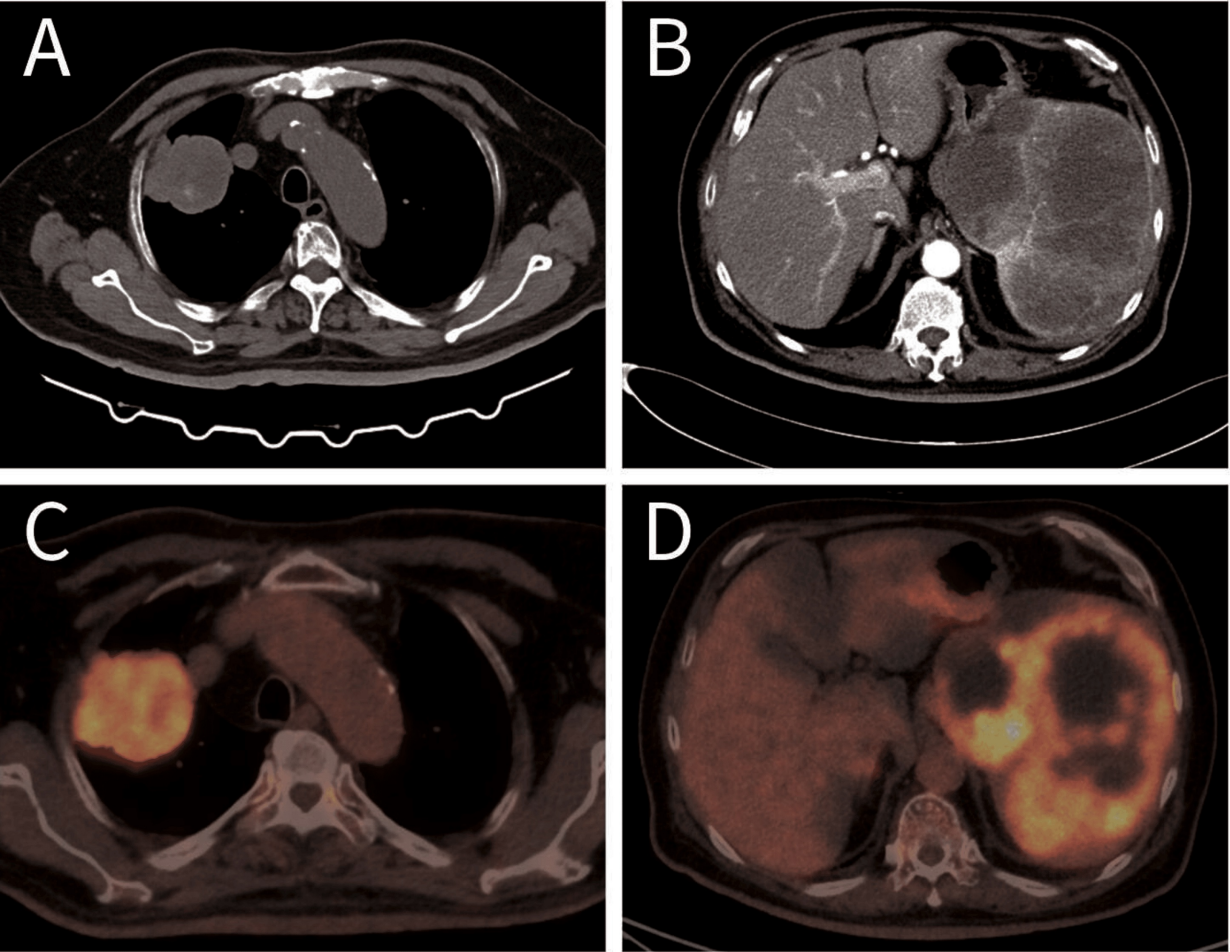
Preoperative axial image of CT and FDG/PET. (A) Soft tissue mass at right upper lung. (B) Several variable-sized heterogeneously contrast-enhanced relatively low-density masses are seen in the enlarged spleen. (C) 18F-FDG-PET/CT image showed (C) FDG-avid mass in the right upper lung, and (D) spleen with central absence of 18F-FDG avidity. FDG, fluorodeoxyglucose.
